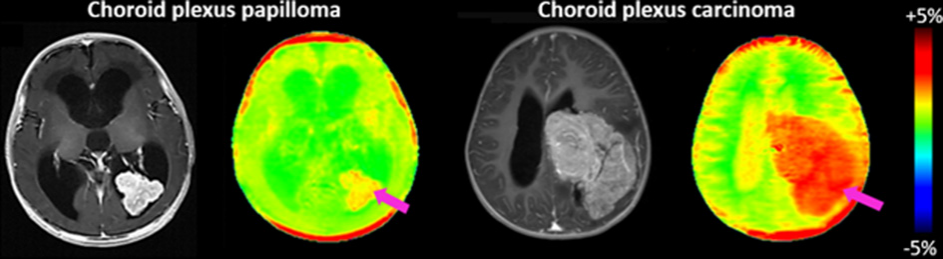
Metabolic imaging. APTw MRI of brain protein concentration. There is moderately increased APTw signal in choroid plexus papilloma and markedly increased signal in choroid plexus carcinoma (pink arrows) compared to the rest of the brain. Normal choroid plexus and CSF in the subarachnoid spaces contain low amounts of protein. Courtesy of Yun Peng, PhD. APTw, amide proton transfer weighted; CSF, cerebrospinal fluid.
